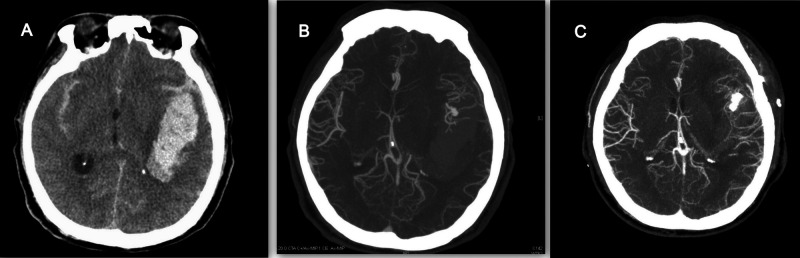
55-year-old female with ruptured left MCA aneurysm. (A) Large left temporal hematoma, but no perisylvian low density, no spot sign, no distal hematoma. (B) CTA revealed M1 bifurcation aneurysm. (C) After successful clipping, there were no delayed progressive mass effect 7 days after operation.
